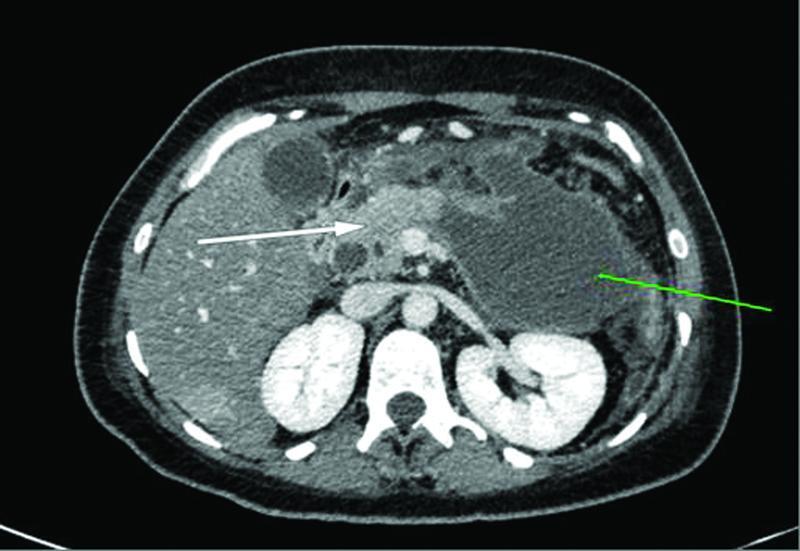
Computed tomography image showing the acute necrotic collection (green arrow) along with diffuse edema of the pancreatic parenchyma (white arrow).
